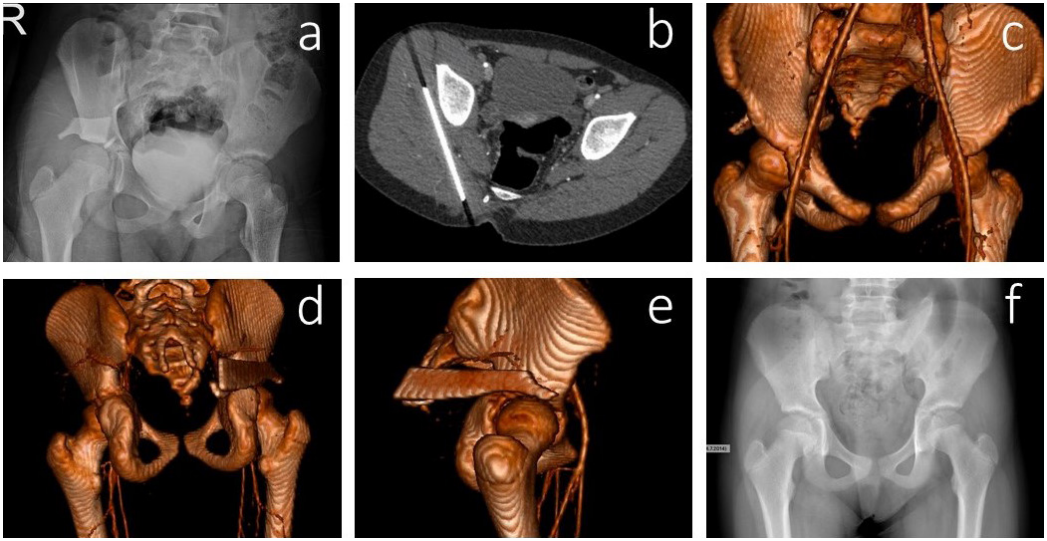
15-year-old female patient with a glass injury on her right hip. Although the FB was clearly visible on the radiogram (a), we initiated a CT of the patient in order to decide on the exact anatomical location of the FB (b); moreover, because of the complex anatomy of the region and morphological variations, an angiography was done to exclude any vital organ injuries, look at the relation to the hip joint, and to plan for her operation (c–e). Under general anesthesia, the glass pieces were removed without any neurovascular injury. Postoperative radiography of the patient (f).
